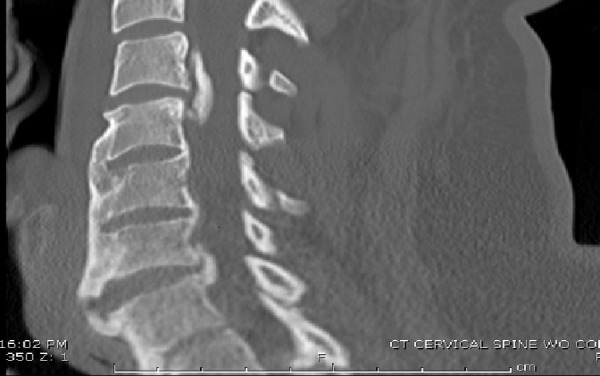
Preoperative sagittal cervical spine CT A focal ossified PLL, anterior autofusion from C4-T1, and a lucency through a C6-7 syndesmophyte
